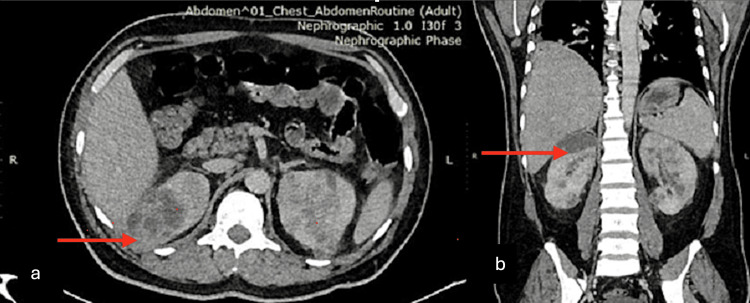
CECT abdomen. Axial (a) and coronal (b) images showing right upper pole renal abscess and multiple other renal abscesses in bilateral kidneys (arrows represent the areas of abscesses) CECT: contrast-enhanced computed tomography
